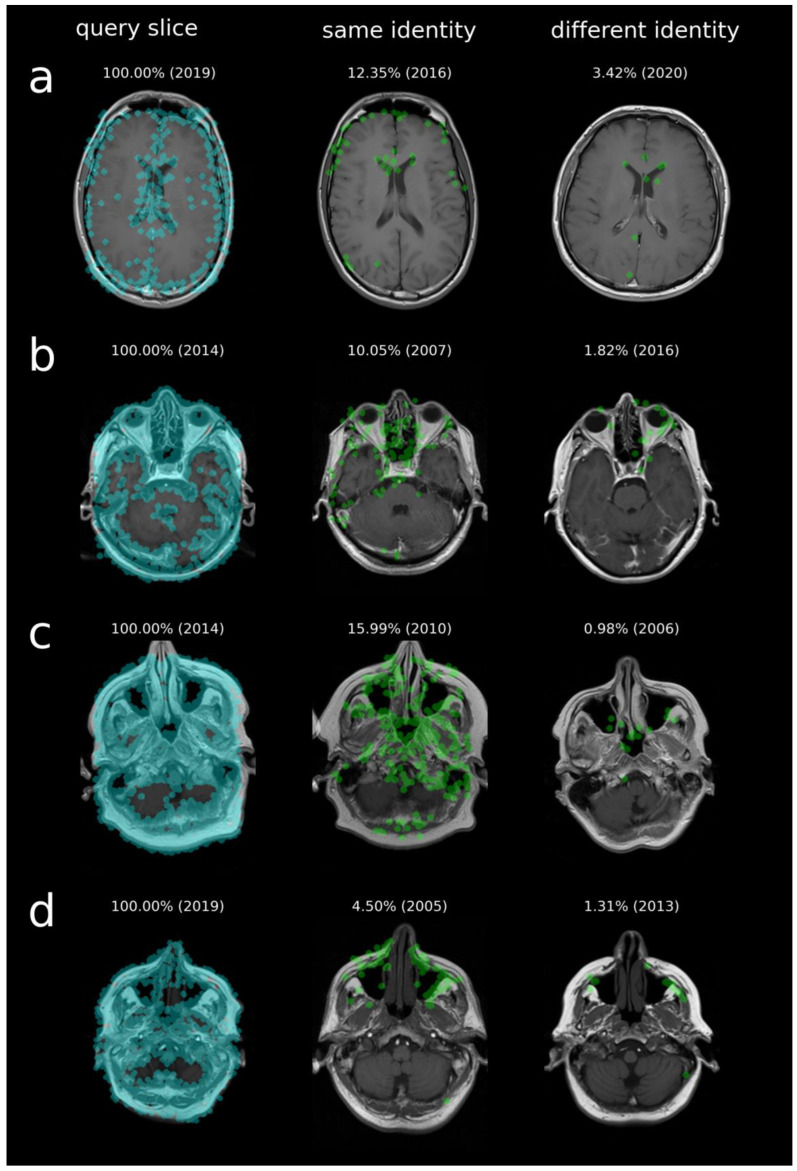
Representative examples of successful identification using individualized anatomical CV features in MRI slices: (a) frontal sinus, (b) ethmoid bone, (c) nasal septum, (d) maxillary sinus. (Left): query slice with detected CV features (blue). (Middle): reference slice from the same individual; (right): best-matching slice from a different individual. Matched CV feature points are shown in green. Despite some anatomical similarities across individuals, the correct reference image is clearly distinguishable, enabling reliable identification. Acquisition year and similarity score (%) are shown.
